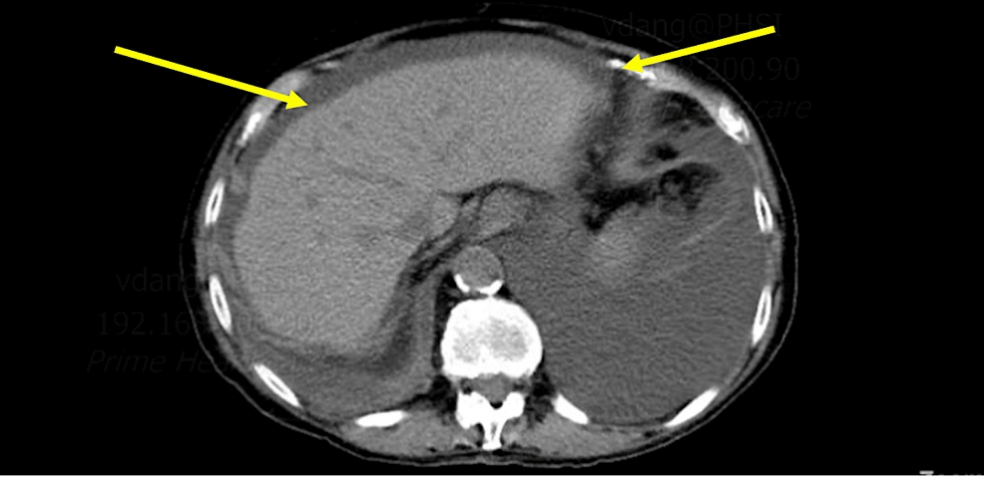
CT scan of the abdomen demonstrating ascites.
